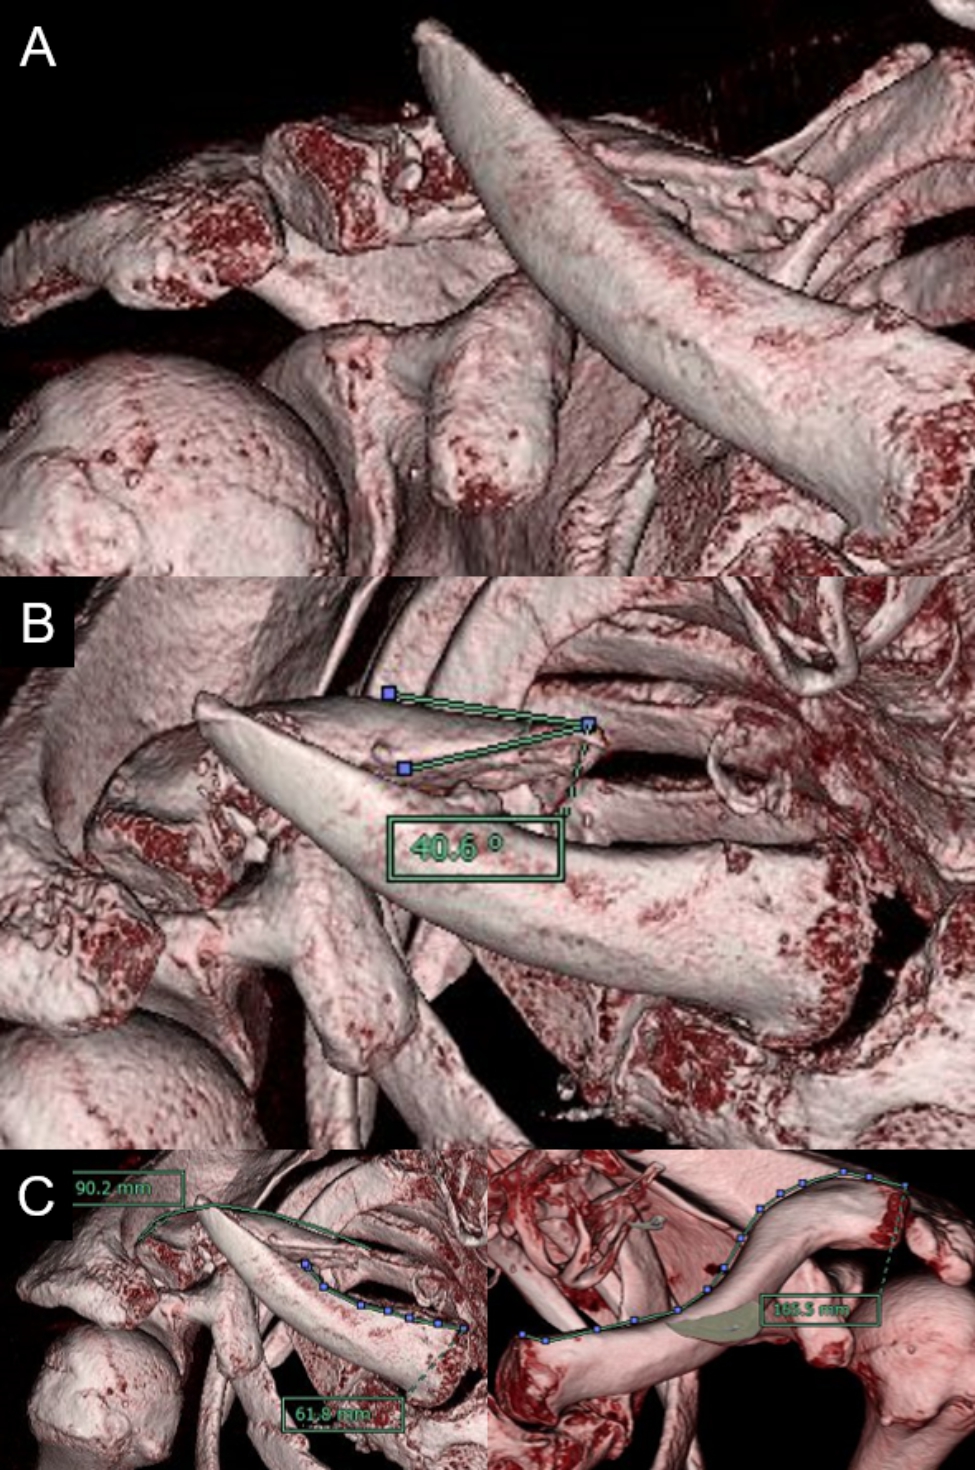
Computed tomography (CT) of the right clavicle 3D-CT demonstrated an atrophic nonunion of clavicle oblique fracture with 40.2° (A and B). The length of the distal and proximal fragments on the affected side was 90.2 mm and 61.8 mm, respectively, while the length of the clavicle on the unaffected side was 165.5 mm (C)
